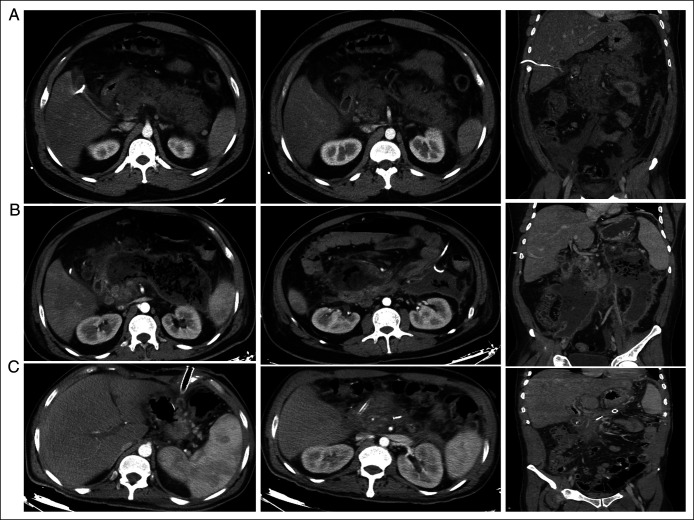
Contrast-enhanced CT imaging timeline. (A) Initial CT scan (day 1, May 29, 2025) demonstrating acute necrotizing pancreatitis with hypoenhancing pancreatic head necrosis and associated portal vein thrombosis, with extensive peripancreatic fluid extending into the paracolic gutters and pelvis. (B) Follow-up CT (June 19, 2025) showing development of a large organized walled-off necrosis at the pancreatic bed extending into both paracolic gutters despite prior drainage interventions. (C) Late follow-up CT (September 28, 2025) demonstrating near-complete resolution of the collection with residual mesenteric fat stranding and no recurrent fluid collection. Only uniform brightness/contrast adjustments were applied. No nonlinear or AI-based image modifications were used. CT, computed tomography.
